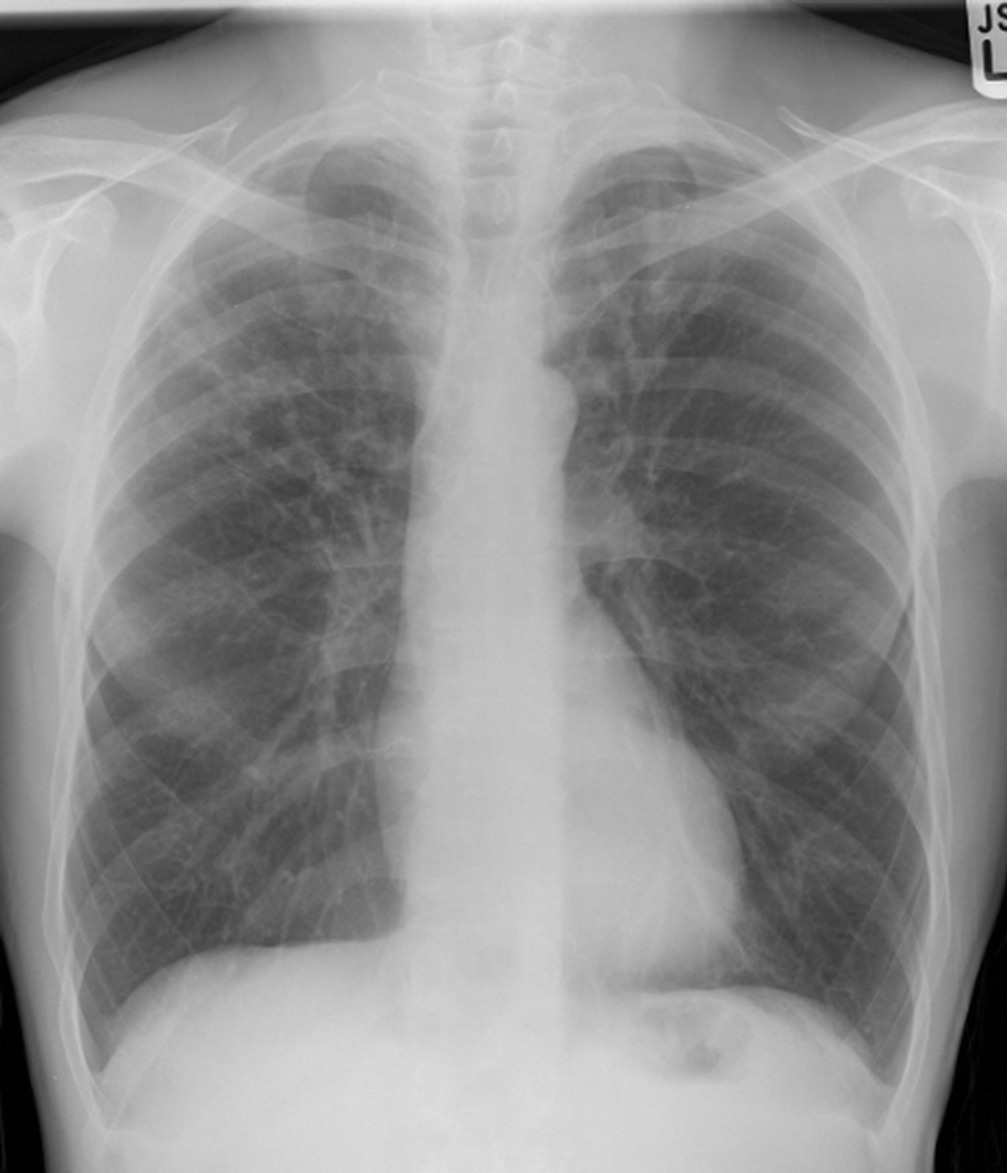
A plain chest radiograph demonstrating infiltrates in both the apices.
